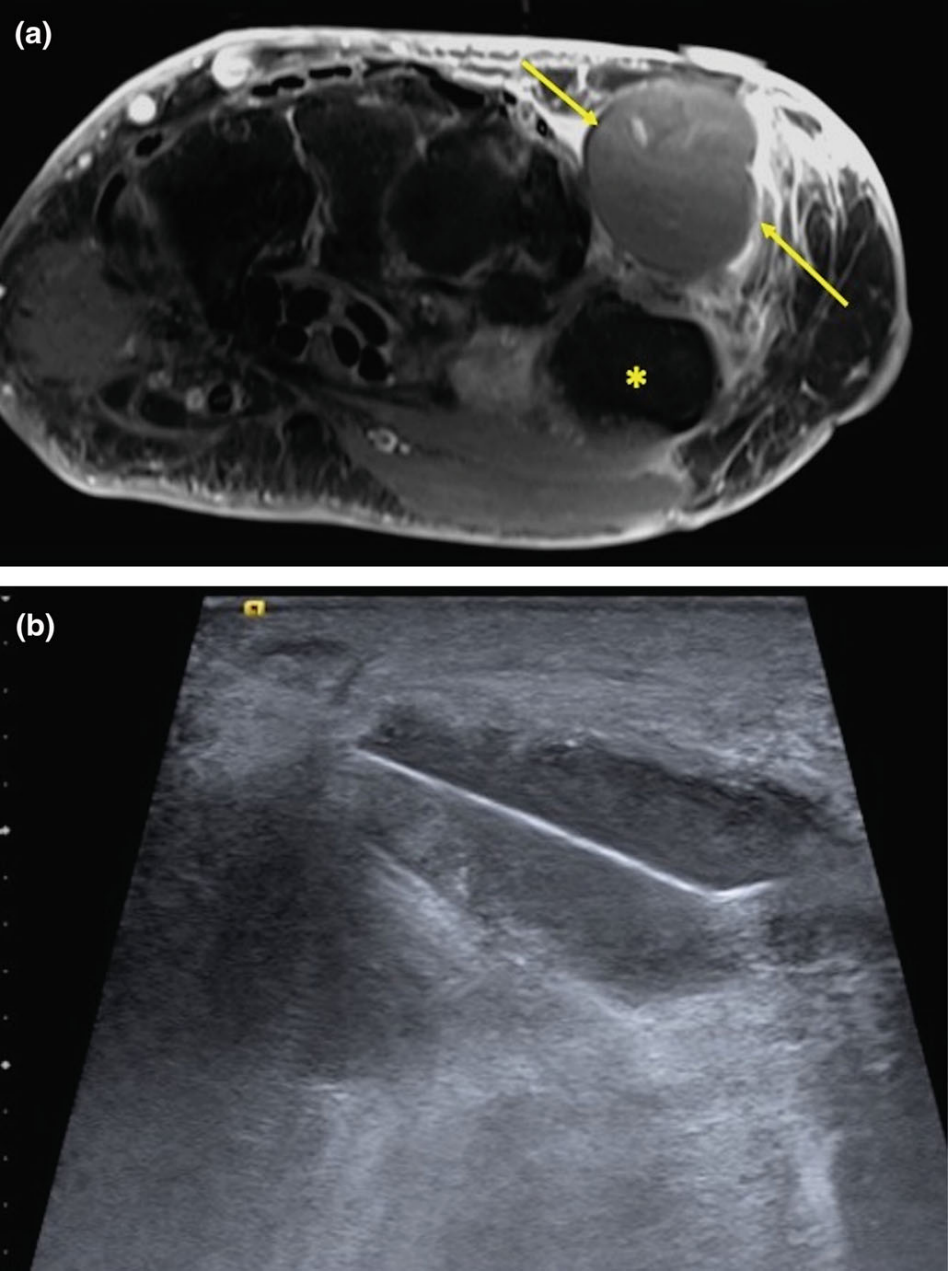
A 67‐year‐old man with a history of previously resected mass from first web space. (a) Contrast‐enhanced, fat‐suppressed, T1‐weighted MRI shows a non‐enhancing cystic mass (arrows) in the first web space, dorsal to the thumb metacarpal (*), and surrounding soft tissue enhancement. (b) Ultrasound‐guided biopsy with a 16‐gauge needle confirmed recurrent carcinosarcoma.
